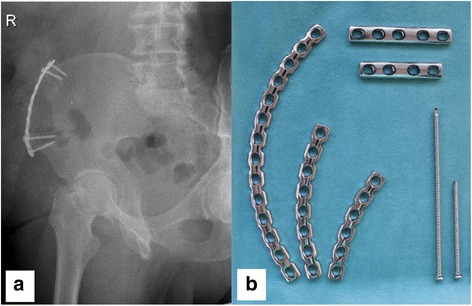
a X-ray (right pelvis ala-view) of a 73-year-old patient 8 years after bone harvesting (vascularized iliac crest) and simultaneous plate osteosynthesis to prevent a fatigue fracture. b Anatomically shaped low profile small fragment plates and cortical screws (optionally angular stable) that can be used to reconstruct the iliac crest following bone graft harvesting
